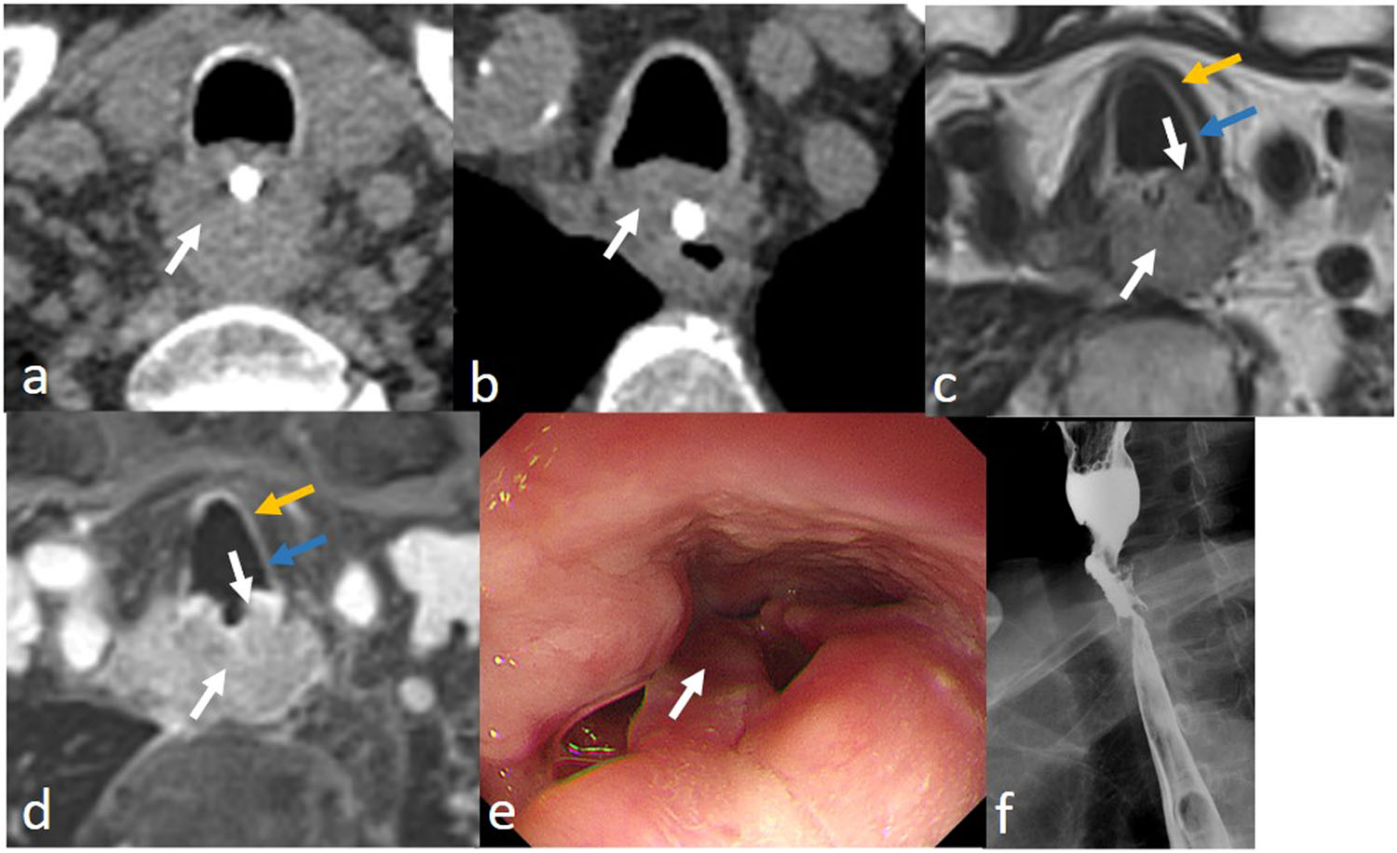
Esophageal fistula in a 62-year-old man. A tumor (white arrow) is poorly defined on CT and tracheal involvement is not confirmed (a, b). MRI clearly delineates that the tumor invades the trachea. The hypointense adventitia (yellow arrow) and hyperintense intima (blue arrow) of the trachea are interrupted in the T2-weighted image (c) and in the contrast-enhanced T1-weighted image (d). The tumor obstructs the entire esophagus, making it impossible for the oesophagoscope or endoscopic ultrasonography to pass through (e). Barium esophagogram shows the esophageal fistula (orange arrow)
